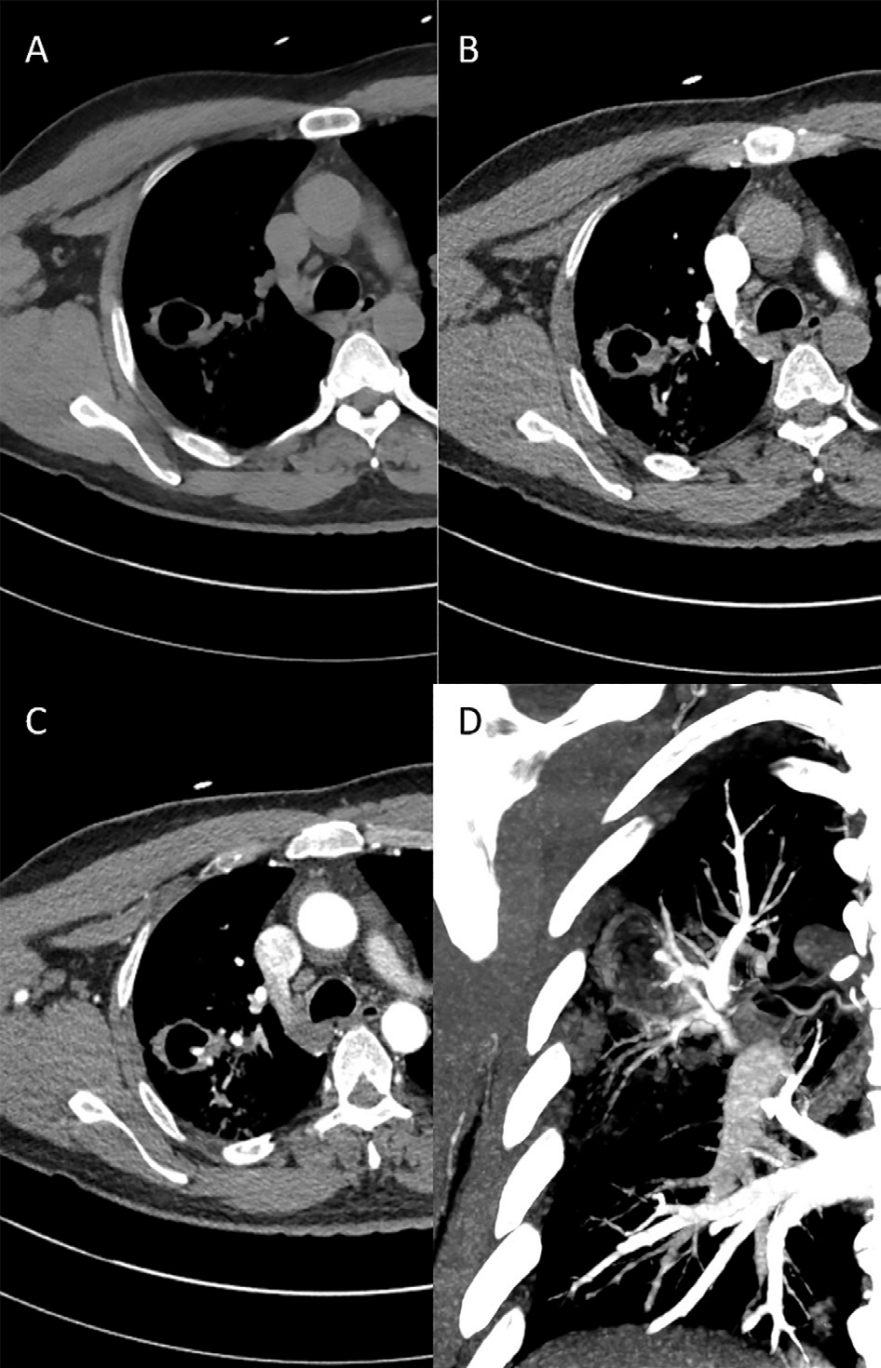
Non-enhanced CT (A), CTA in pulmonary phase (B) and CTA in systemic arterial phase (C) of the cavernous lesion in the right upper lobe. (D) shows the 30 mm coronal MIP images of the systemic arterial phase (C).
